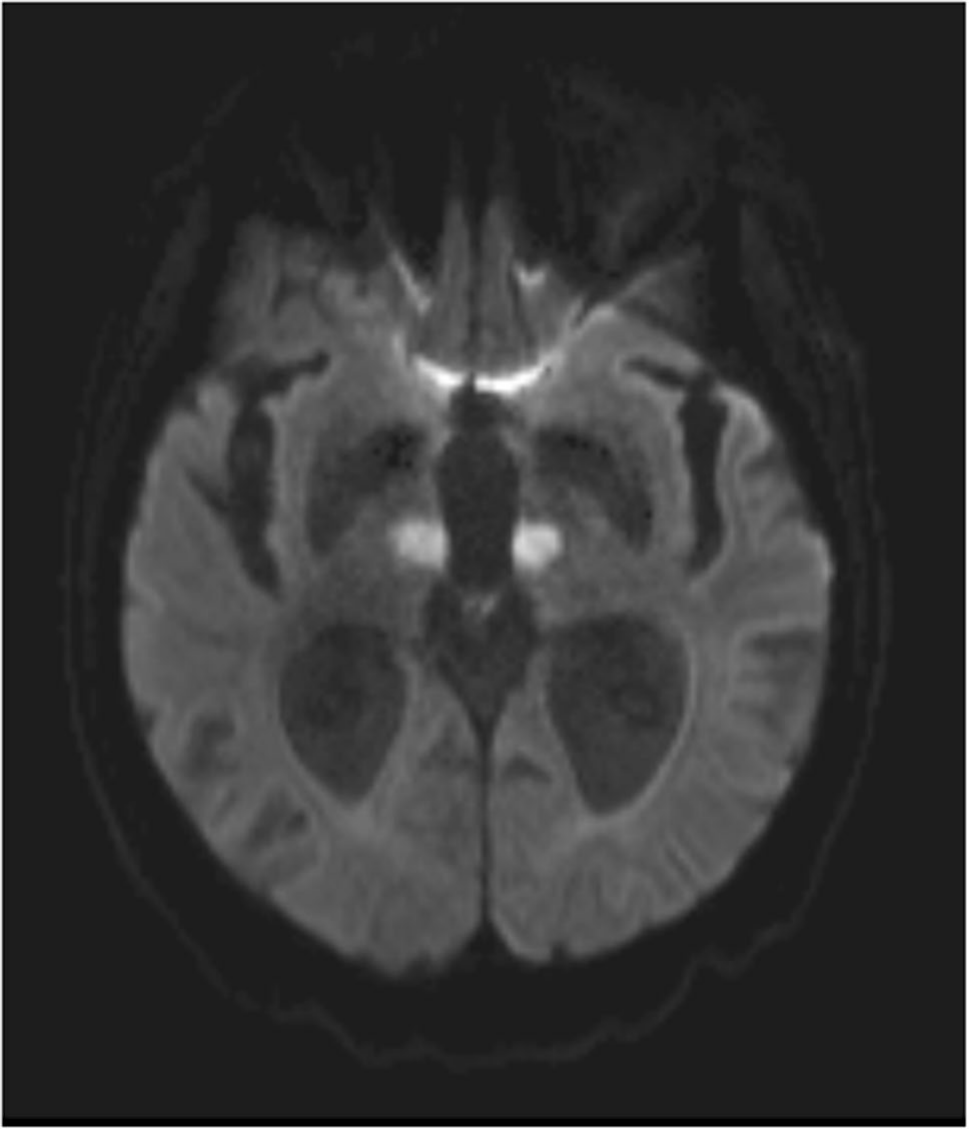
Example of bilateral, paramedian ischemic lesion in the thalamus (DWI imaging)
